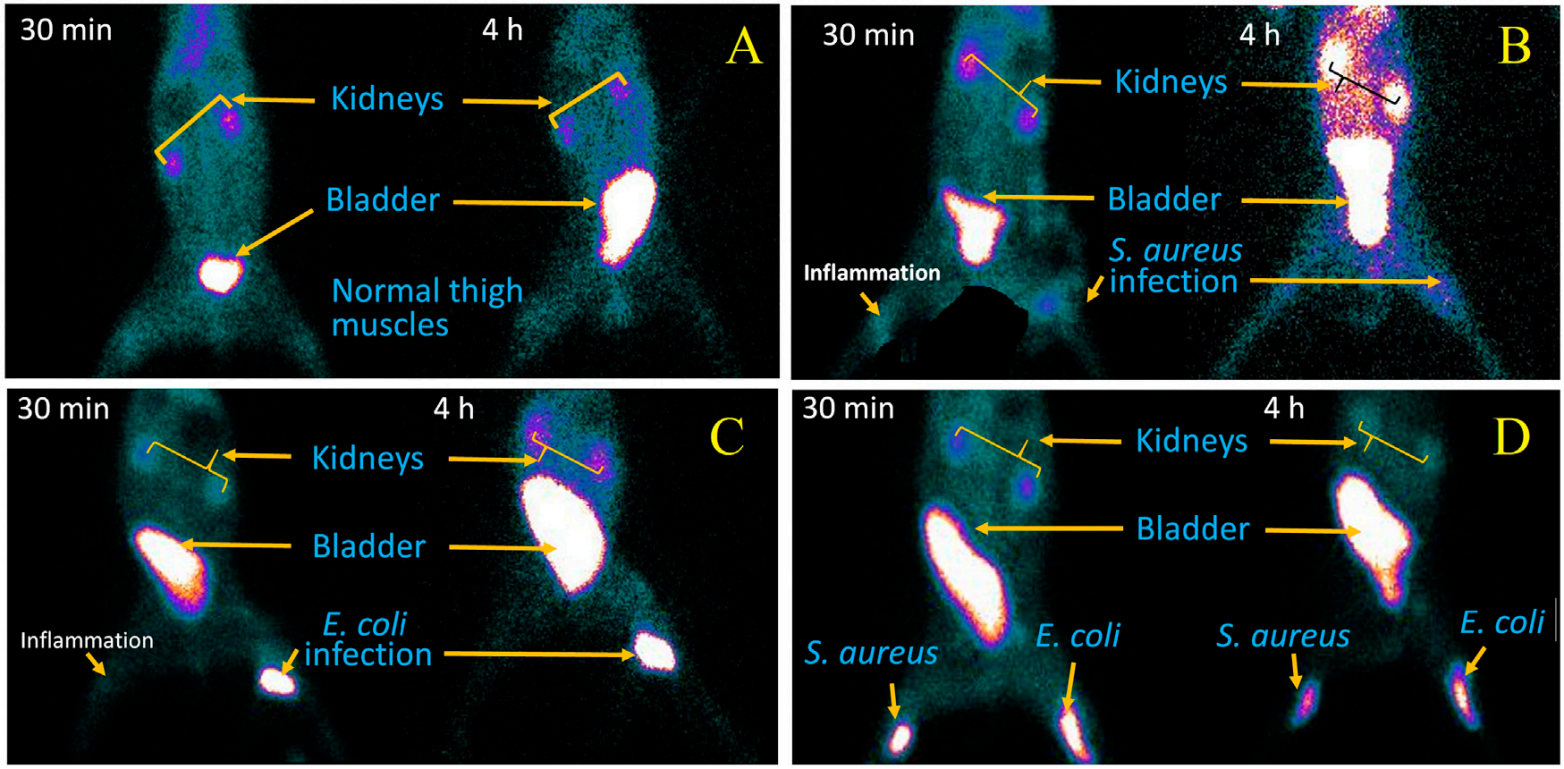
SPECT gamma camera scintigraphy images, at 30 min and 4 h post injection of ^99m^Tc-ertapenem radiopharmaceutical, of: (A) healthy rabits, (B)
